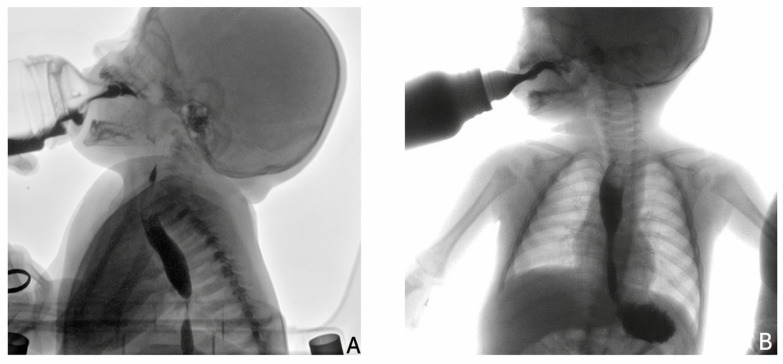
Contrast esophagography in a 5-month-old infant with congenital esophageal stenosis. (A) Lateral view showing marked narrowing of the mid-distal esophagus with mild proximal dilatation. (B) Anteroposterior view confirming reduced passage of contrast through the stenotic tract. The stenotic segment measured approximately 18 mm in length on MRI.
